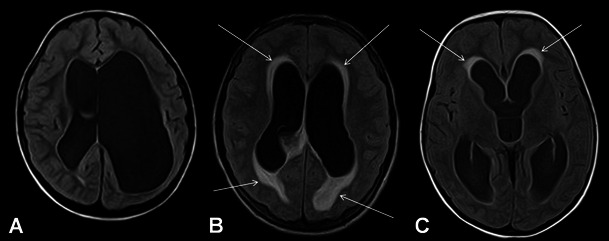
Axial FLAIR images of three different patients with hydrocephalus. In the first patient with chronic compensated hydrocephalus, lack of periventricular CSF resorption is seen (A). In the second patient, periventricular hyperintensity consistent with interstitial oedema due to acute decompansated hydrocephalus is demonstrated (*arrow*, B). Periventricular caps seen in middle aged adults should be differentiated from decompansated hydrocephalus (*arrows*, C)
